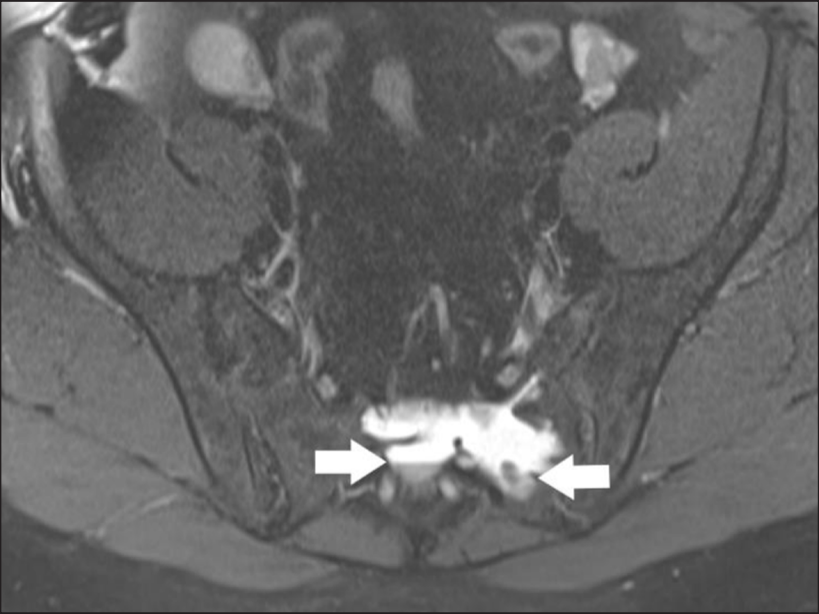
A 43-year-old female with aneurysmal bone cyst. Axial fat saturated T2-weighted MR image shows a lobulated cyst with multiple fluid-fluid levels (arrow). Remark the sedimentation of blood cells caused by slow flow of the blood in the cystic spaces of the tumour.
